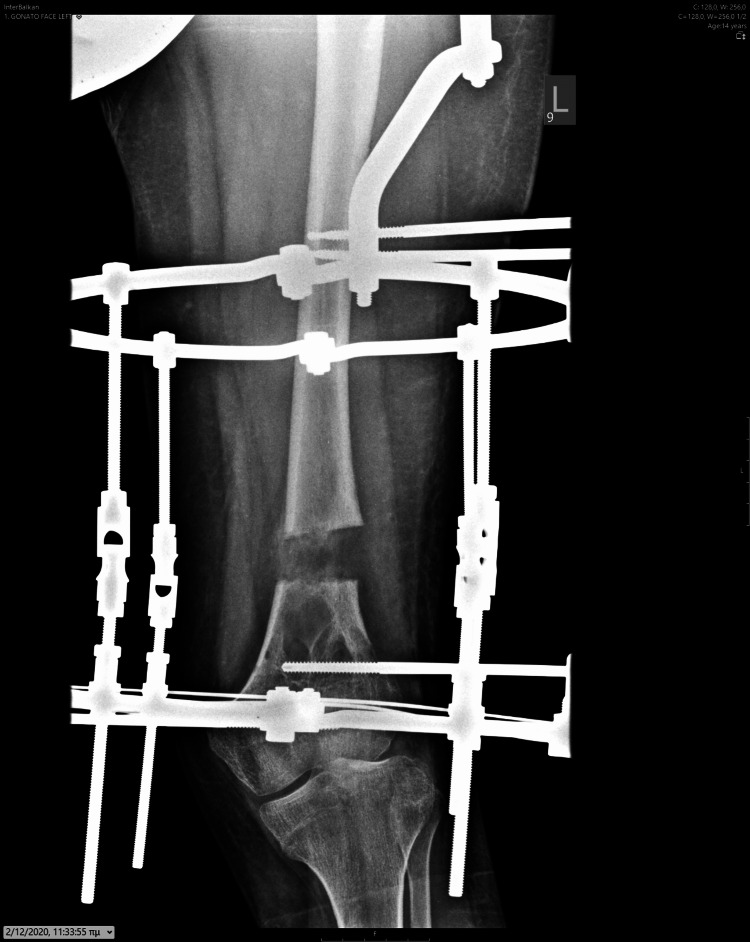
Antero-posterior X-ray of the femur, depicting the asymmetrical lengthening achieved through the Ilizarov device.
